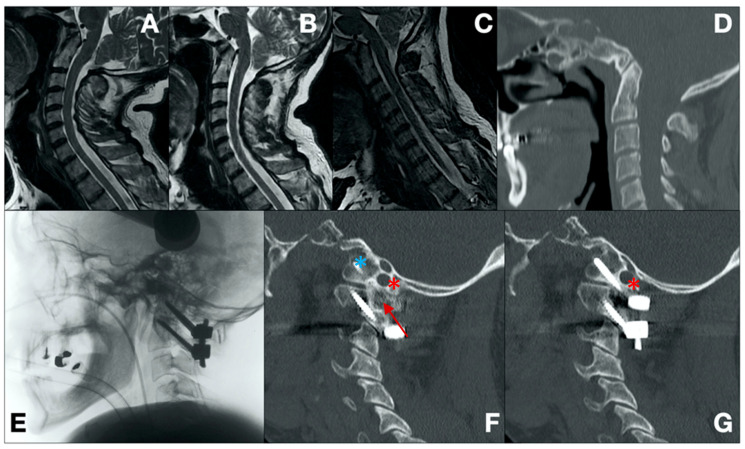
Pre-operative T2-weighted extension, neutral, and flexion sagittal MRI scans, respectively (A–C). Pre-operative sagittal CT scan (D). Intra-operative radiographic acquisition (E). 30-month follow-up sagittal CT scans demonstrating correct alignment, arthrodesis achievement (red arrow), and correct screw insertion avoiding critical neurovascular structures (red asterisk for vascular structures and blue asterisk for neural structures) (F,G).
